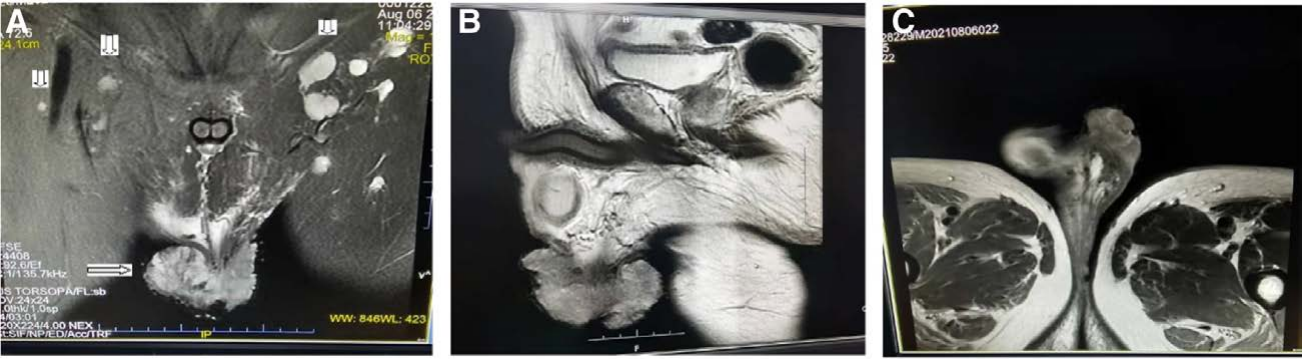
MRI of the tumor. (A) Coronal plane, (B) sagittal plane, and (C) horizontal plane showed a malignant scrotal mass with bilateral inguinal lymph node metastasis. MRI = magnetic resonance imaging.
